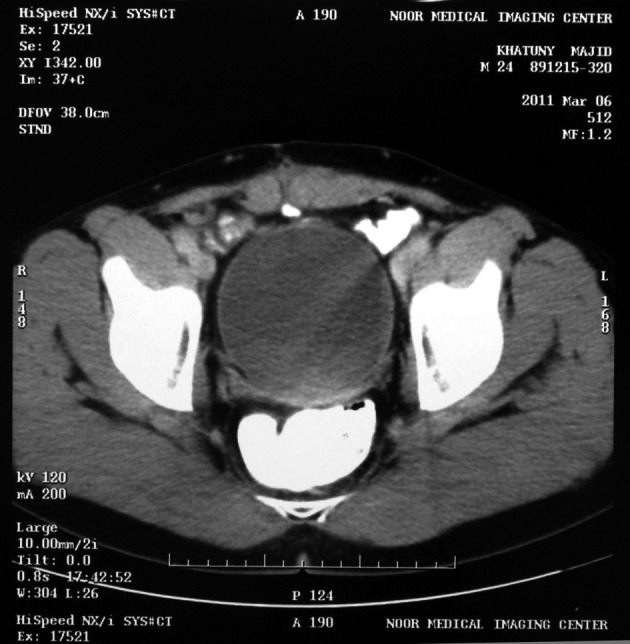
Normal CT Scanning After Two Years Follow-up.
